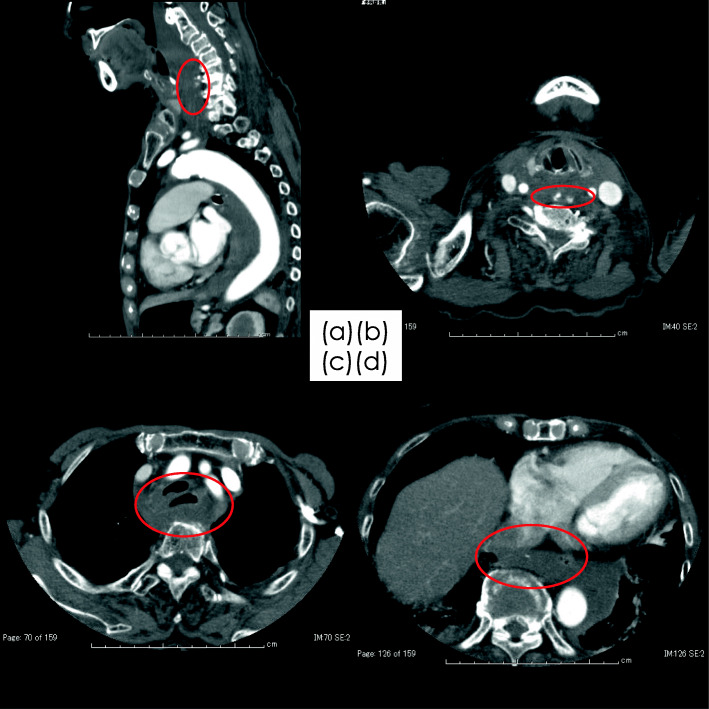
Computed tomography scans show retropharyngeal hematoma and extravasation (circled area) (a, b). The hematoma extends to the posterior mediastinum and compresses the trachea (c, d)
